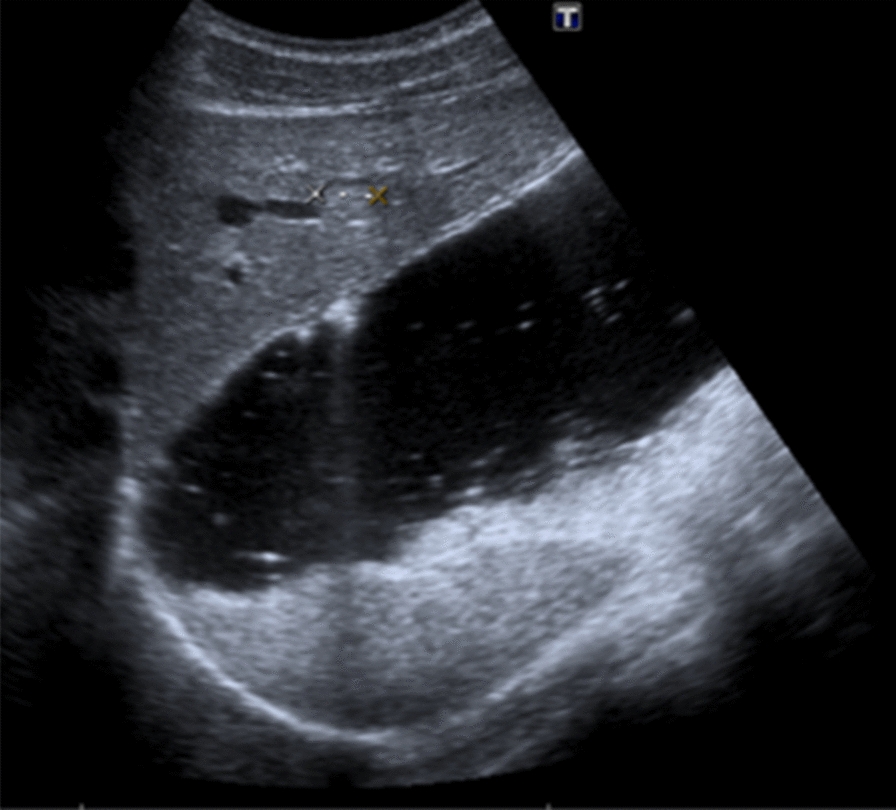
Abdominal ultrasound shows a 1.3 cm lesion in liver segment 2, adjacent to the left suprahepatic branch, isoecogenic with a slightly more hypoecogenic halo
